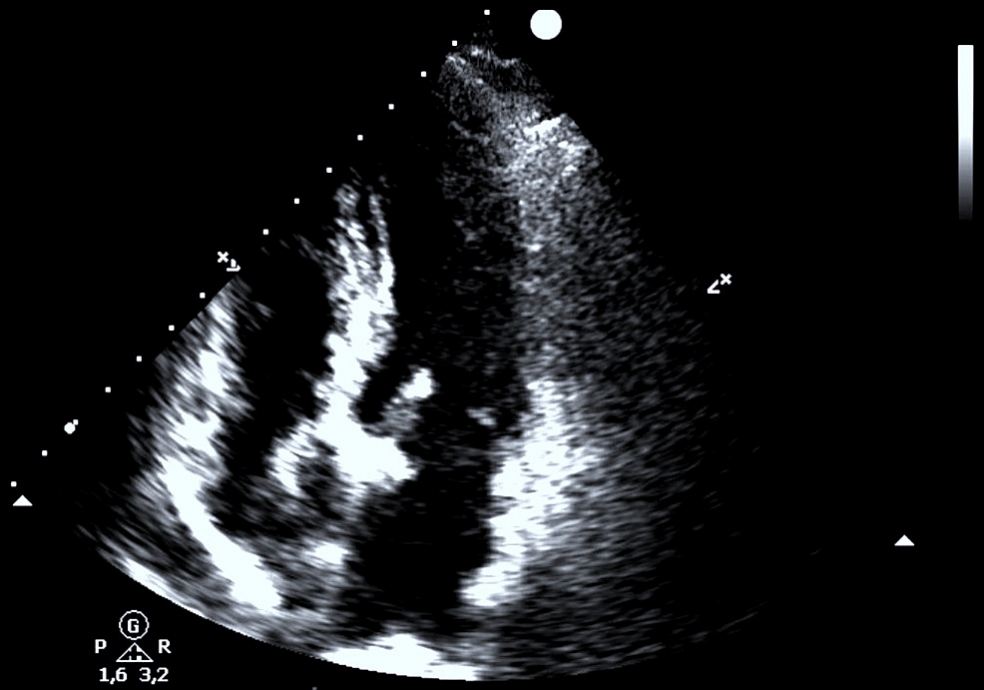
Postoperative TTE showing the bioprosthetic aortic valve with the resolution of mitral SAM TTE: transthoracic echocardiogram; SAM: systolic anterior motion.
